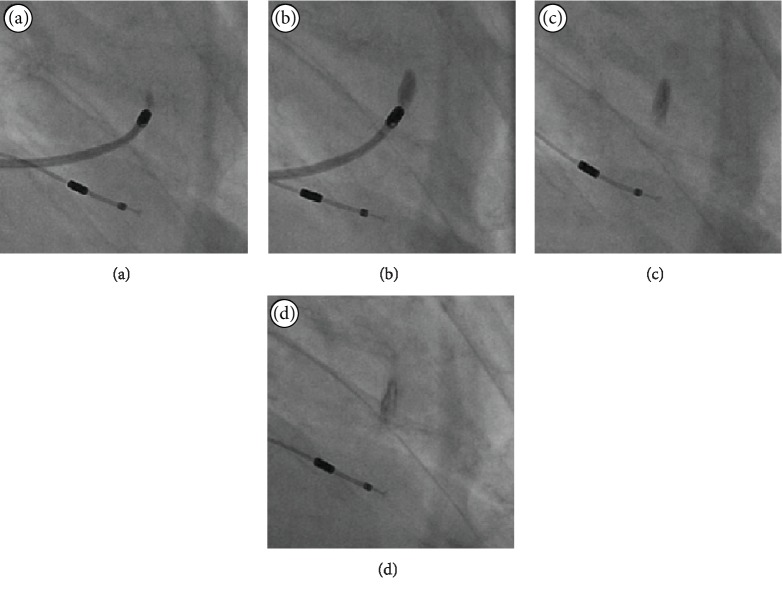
NOGA injection dispersion pattern. X-ray images demonstrate the immediate dispersion pattern of an injected solution using the NOGA system, in this instance, iodine contrast. Images (a) and (b) depict the start and end of injection over approximately one minute, while images (c) and (d) after 2 and 3 minutes.
